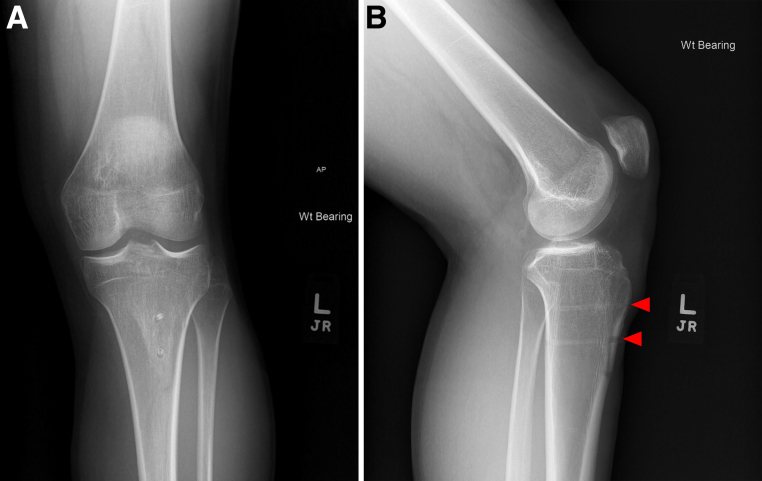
Representative (A) anteroposterior and (B) lateral postoperative radiographs of tibial tubercle osteotomy fixation showing osseous union with an osteotomy line and shadow of 2 radiolucent biointegrative screws (red arrowheads) at 4.6 months.
